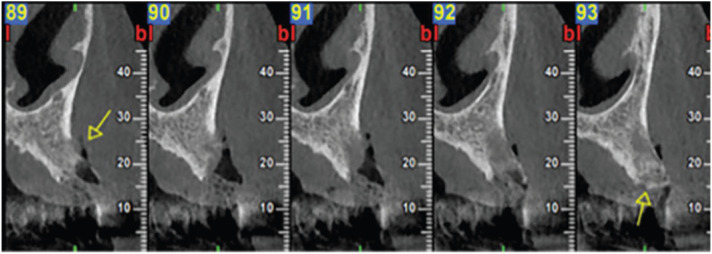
Imaging examination. Cone beam computed tomography scan showing osteolytic lesion with ill-defined borders and buccal and palatal perforation in the left maxillary alveolar bone between central incisor and second premolar.
